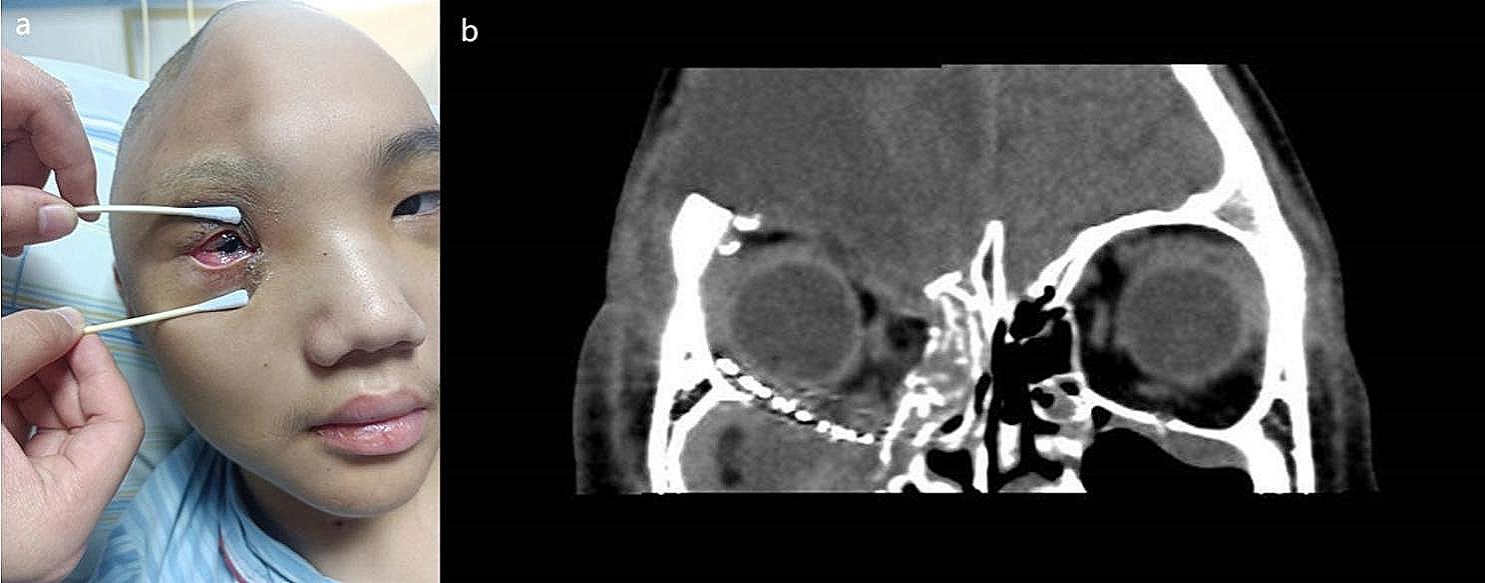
Postoperative examination. (a). Bedside examination revealed right eye conjunctival oedema, a pupil diameter of 2.5 mm, sluggish light reflex, and limited eye movement. (b). Computed tomography showed the titanium mesh in a satisfactory position with no entrapment of orbital tissues
